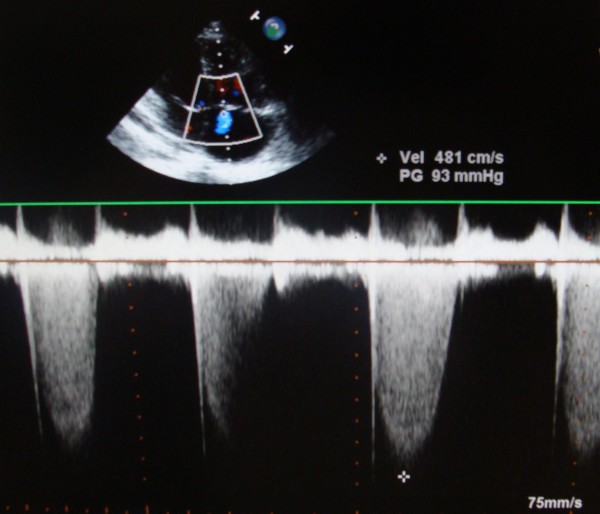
Severe tricuspid regurgitation and important pressure gradient (93 mmHg), assessed by continuous wave Doppler in apical four-chamber view of transthoracic echocardiography.
